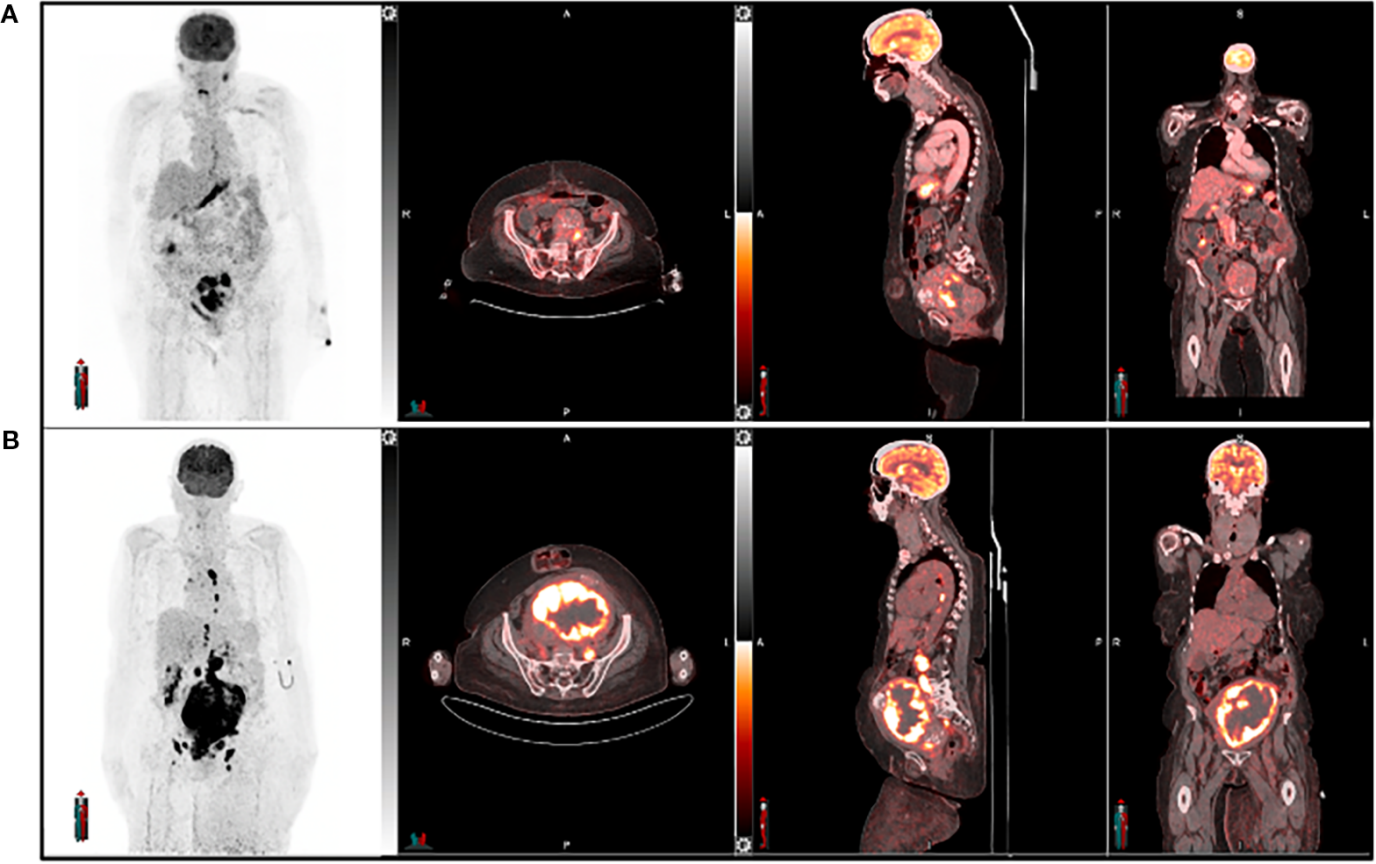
A 64-year-old female with endometrial cancer underwent [^18^F] FDG PET imaging for both diagnosis and management. The pre-treatment scan (A) was used for initial assessment, while the post-treatment scan (B) evaluated treatment response six weeks after therapy completion. Follow-up imaging revealed an increase in the size of the primary tumor and nodal metastatic lesions, along with heightened tracer uptake, suggesting disease progression or treatment failure.
